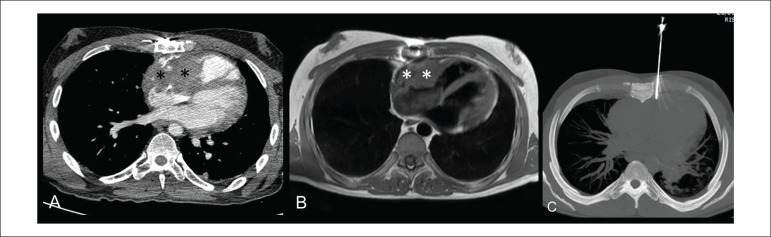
A) Axial contrast-enhanced CT image showing heterogeneous mass (asterisks)
